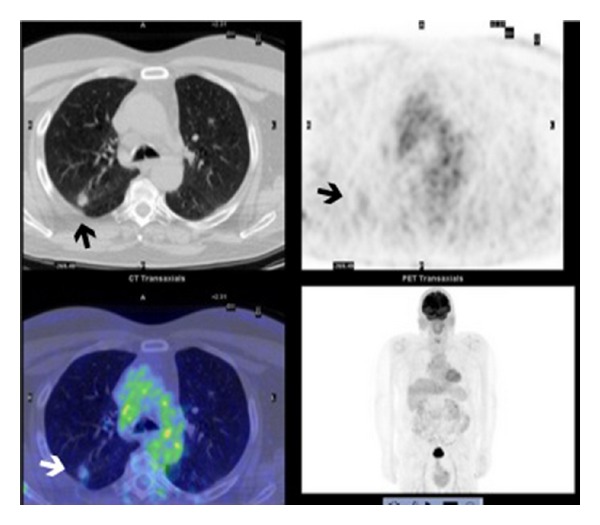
Faintly ^18^F-FDG uptake of the right pulmonary upper lobe—SUVmax 1.3 (arrow).
